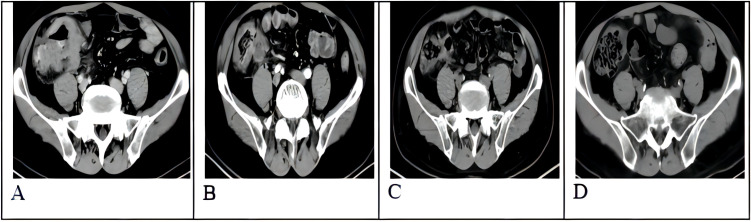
CT scan of tumor mass changes before and after infusion of CAR-T cells. (A) CT scan before infusion of CAR-T cells. (B) +28 days CT scan after infusion of CAR-T cells. (C) +75 days CT scan after infusion of CAR-T cells. (D) One year CT scan after infusion of CAR-T cells.
